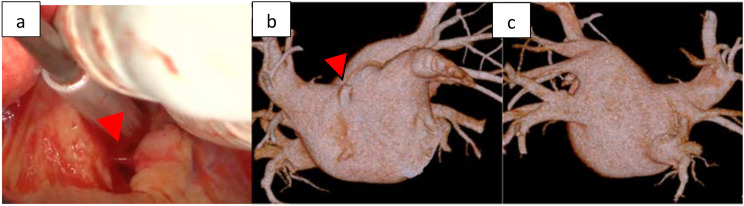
Case 3. a: Bleeding point of a left atrium diverticulum. b,c: Perioperative computed tomography showing a left atrial diverticulum (red arrow). b: Anterior left atrium, c: Posterior atrium
